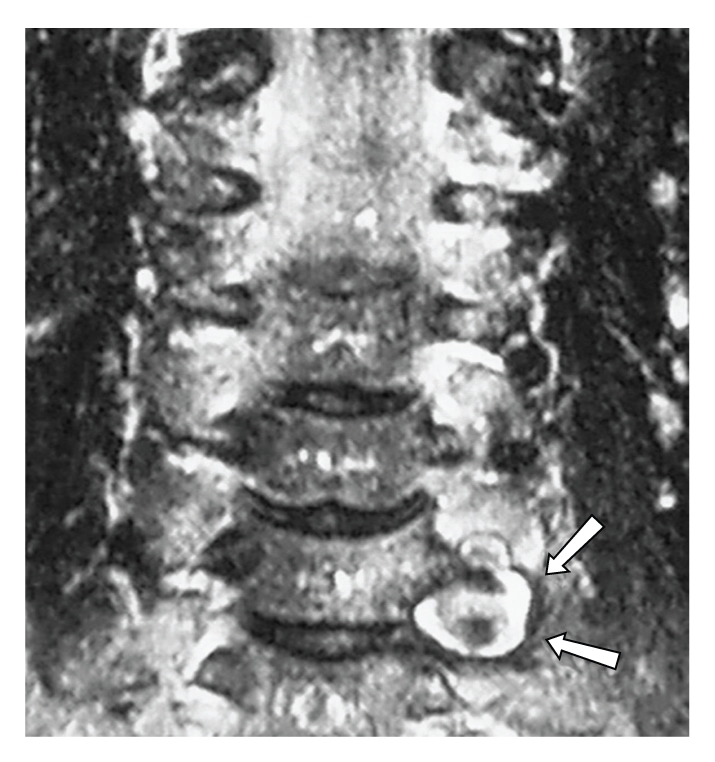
Coronal T2-weighted image of the cervical region; arrows point the aneurysm.
